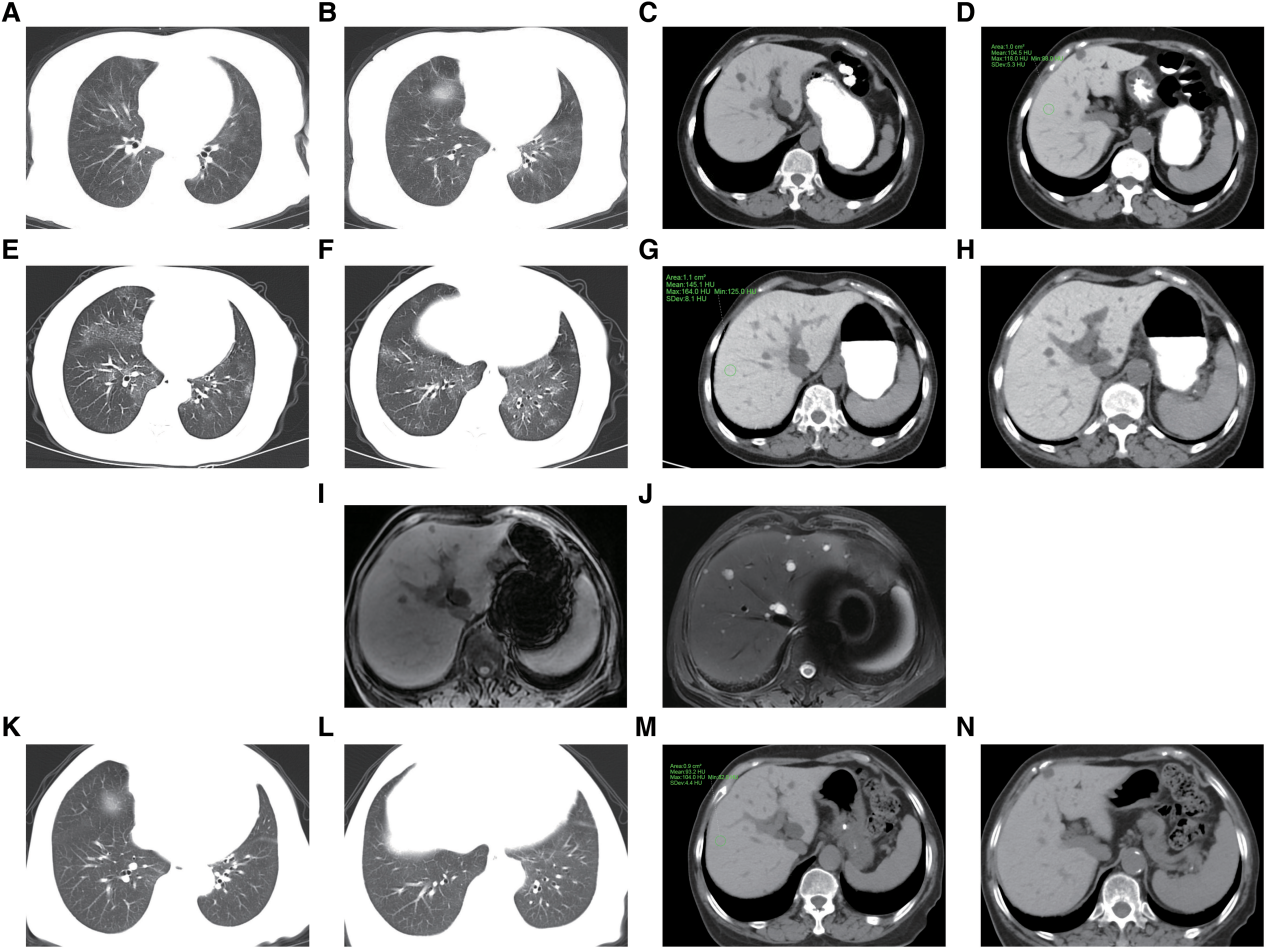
Imaging changes before (2022.02.14), at the onset of organ damage (2023.08.16), and 6 months (2024.02.09) after discontinuation of the amiodarone. (A–D) After 1 year (2022.02.14) of treatment with oral amiodarone (0.2 g/day), diffusely distributed ground-glass density shadows were seen in both lungs, with perifollicular hyperdense shadows, which were considered to be organizing pneumonia; the liver was hyperdense with a CT value of about 104 hu. (E–J) After 2 years (2023.08.16) of oral treatment with amiodarone, chest CT showed diffuse ground-glass shadows and fine reticular shadows in both lungs, with thickening of the interlobular septum, which was more significant than before; the density of the liver was markedly higher than before, with a CT value of about 145 HU. MRI of the liver showed the hepatic parenchyma was isointense on T1WI, and isointense on the fat-suppressed sequence of T2WI. (K–N) After stopping taking amiodarone for 6 months (2024.02.09), most of the lung lesions disappeared, and the density of the liver decreased, the CT value was about 93 HU.
